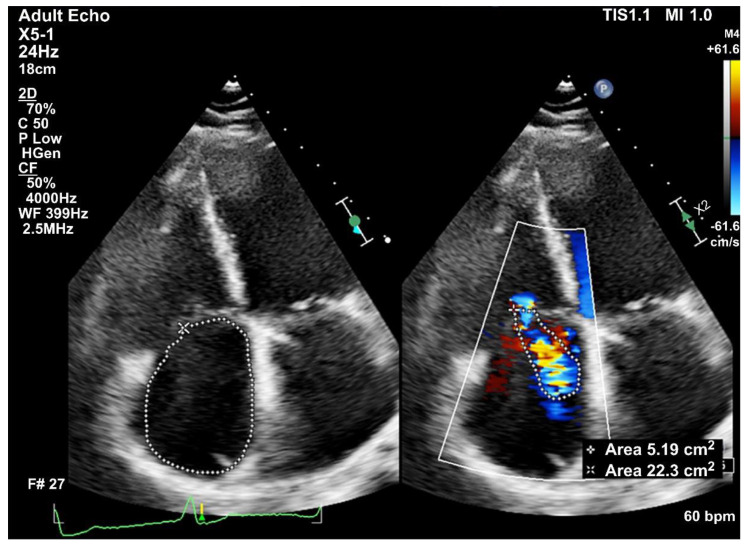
Tricuspid regurgitation (TR) jet area-to-right atrium ratio in the end-systolic phase measured using two-dimensional transthoracic echocardiography. The left panel shows the right atrium area (dot line = 22.3 cm^2^). The right panel shows the TR jet area (dot line = 5.19 cm^2^). The TR jet area-to-right atrium ratio = 23.3% (>20% is considered significant TR).
